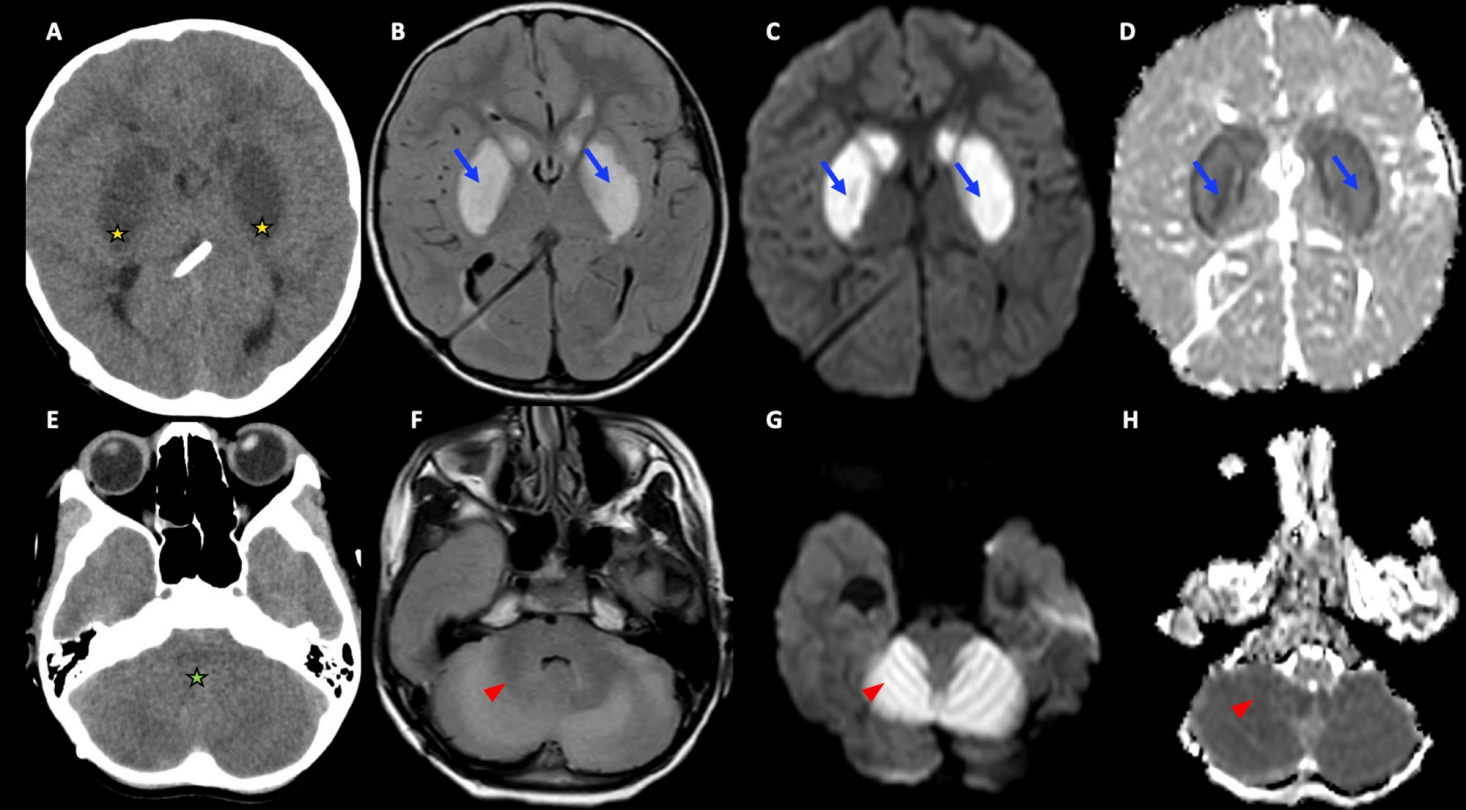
Initial CT and MRI Imaging Findings Images 1A and 1E: CT images demonstrated hypoattenuation in the basal ganglia (gold stars) and cerebellum (green star). Images 1B, 1C, 1D: T2 fluid-attenuated inversion recovery (FLAIR), isotropic diffusion-weighted, and apparent diffusion coefficient (ADC) map images demonstrated corresponding areas of diffusion restriction in the basal ganglia (blue arrows) suggestive of hypoxic-ischemic or toxic-metabolic injury. Images 1F, 1G, 1H: T2 FLAIR, isotropic diffusion-weighted, and ADC images demonstrated diffusion restriction in the cerebellum (red arrowheads) suggestive of hypoxic-ischemic or toxic-metabolic injury. These images were acquired immediately after the patient was noted to be unresponsive following his procedure.
